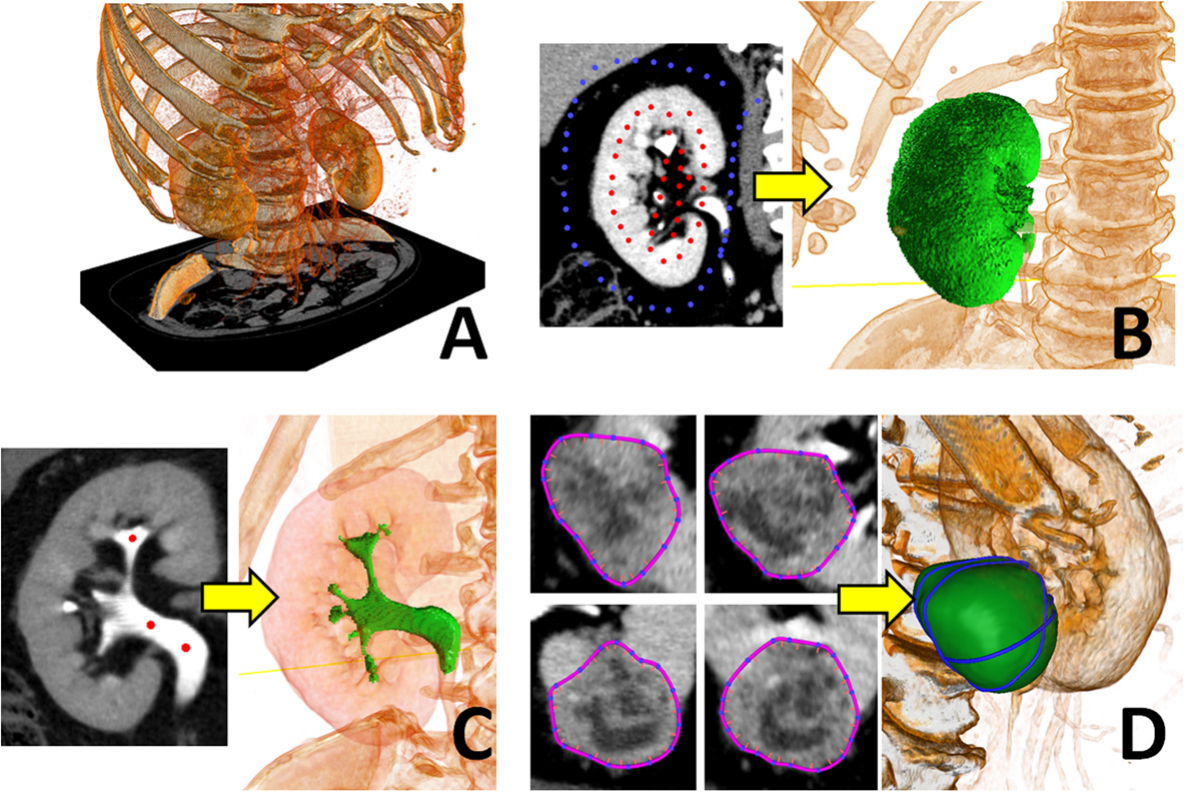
Segmentation of CT image. From an input 3D CT image (A), we segmented the three regions by different methods. (B) The kidney region was segmented by the graph cut method [8,9]. We specified several foreground (red) and background (blue) points on a cross section and the method computed the kidney region from the points. (C) The urinary tract region was segmented by region growing [7]. The method grew the urinary tract region from several seeds (red points). (D) The tumor region was segmented by the contour-based method [11]. The method computed the boundary surface from specified multiple contours.
